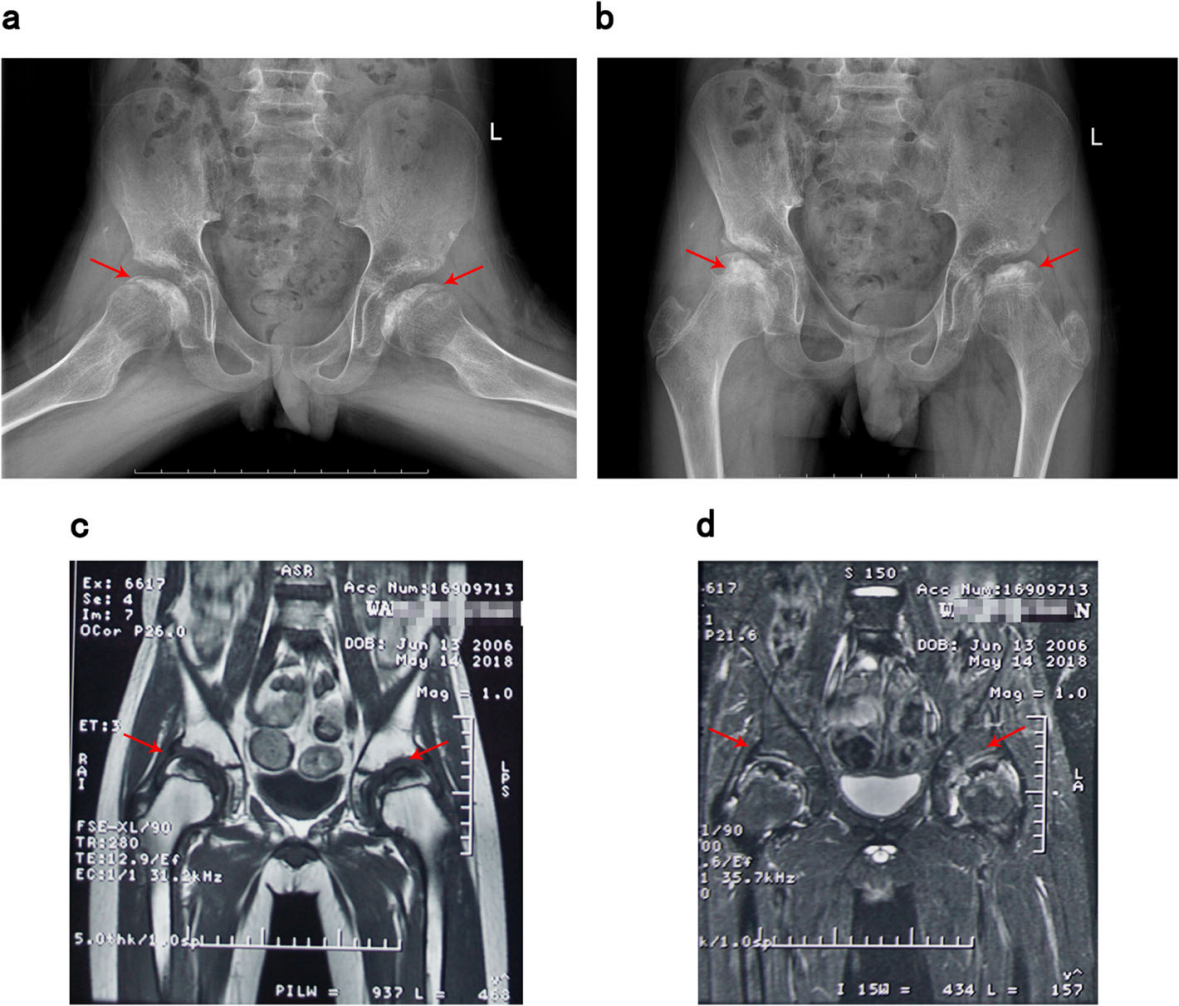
Patient’s bilateral hip X-ray and magnetic resonance imaging (a-b: hip X-ray imaging; c-d: hip magnetic resonance imaging. The arrows point out pathological structures in examinations, indicating bilateral necrosis of the femoral osteoepiphysis)
